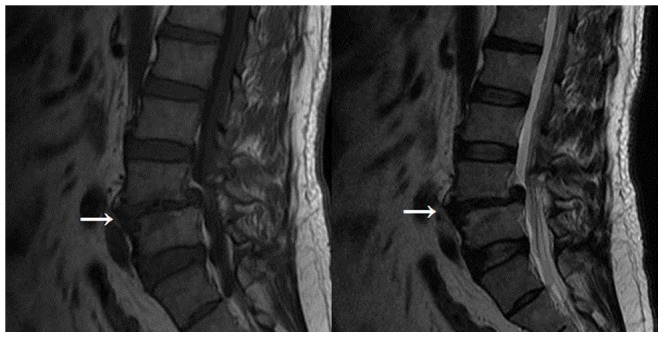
Modic type I changes (indicated by arrow) appear hypointense on. T1-weighted and hyperintense, on T 2 weighted images.
